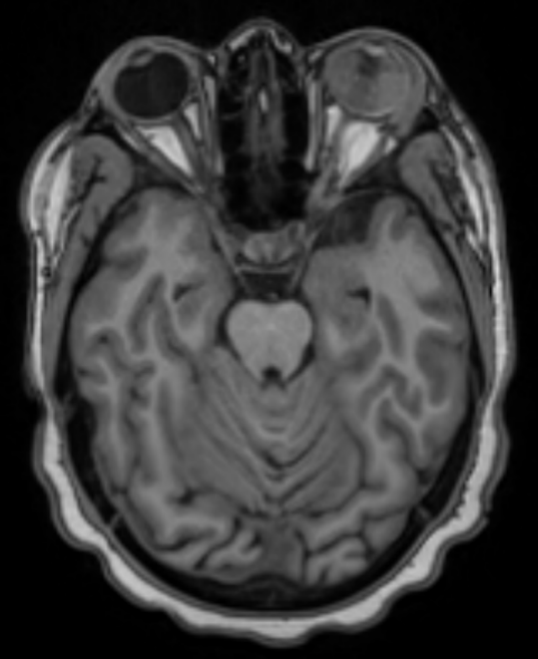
Orbital and cranial MRI revealed an intraocular mass with gadolinium enhancement and lacrimal gland enlargement. No signs of CNS metastasis were found.
